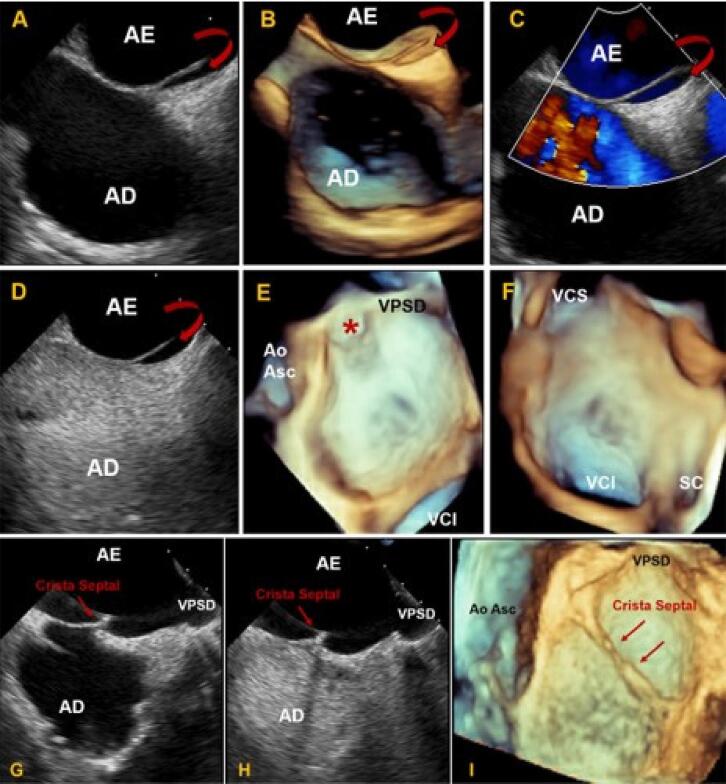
Imagens ecocardiográficas transesofágicas mostrando estrutura semelhante a bolsa em SIA em visão bicaval bidimensional (A) e tridimensional (B) com o orifício da bolsa septal esquerda (setas curvadas). (C e D) Doppler em cores (C) e uso de microbolhas de salina agitada (D) mostrando ausência de shunt septal. Imagens ecocardiográficas em (E e F) Visão “en face” do SIA, de perspectiva atrial esquerda (E) e direita (F). Imagens ecocardiográficas transesofágicas de SIA com presença de crista septal. (G) Visão bidimensional bicaval mostrando imagem de crista septal (seta). (H) Visão bidimensional bicaval mostrando integridade do SIA durante injeção de contraste de salina agitada. (I) Visão en face (3D Zoom) do SIA de perspectiva atrial esquerda mostrando morfologia ecocardiográfica tridimensional da crista septal (setas). AD: átrio direito; AE: átrio esquerdo; Ao Asc: aorta ascendente; SC: seio coronariano; SAI: septo interatrial; VCI: veia cava inferior; VCS: veia cava superior; VPSD: veia pulmonar superior direita.
